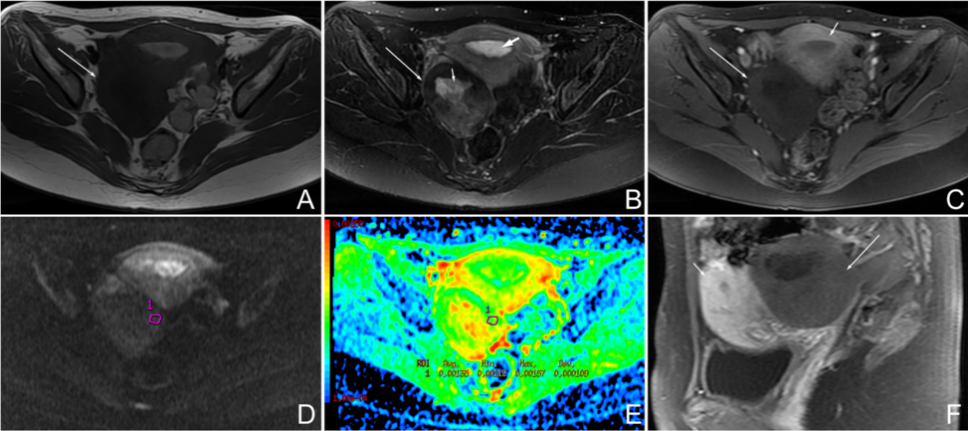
Female, 27 years, fibrothecoma larger than 6 cm on the right ovary. a Axial T1WI showed a solid mass with isointensity (*arrow*). b On axial fat saturated T2WI, the mass (*arrow*) was hypointensity compared with the myometrium. Hyperintensity in the mass represented degenerative changes (*short arrow*). This tumor was accompanied with endometrial hyperplasia (*bold arrow*). c On axial contrast-enhanced fat saturated T1WI, the mass (*long arrow*) revealed minor enhancement, weaker than the myometrium (*short arrow*). d On DWI, the mass appeared as a slightly high signal (grade 1). e On ADC map, the mean ADC value was 1.35 × 10^−3^ mm^2^/s. f On sagittal contrast-enhanced fat-saturated T1WI, the manifestation was similar to that shown in Fig. 2c
